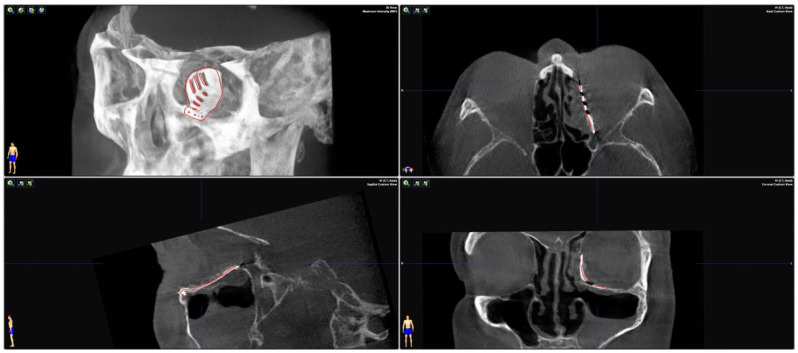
Merging the pre-operative planning CT data with the post-operative CBCT scan confirms correct positioning as the virtually planned patient-specific implant co-localizes with the inserted implant.
